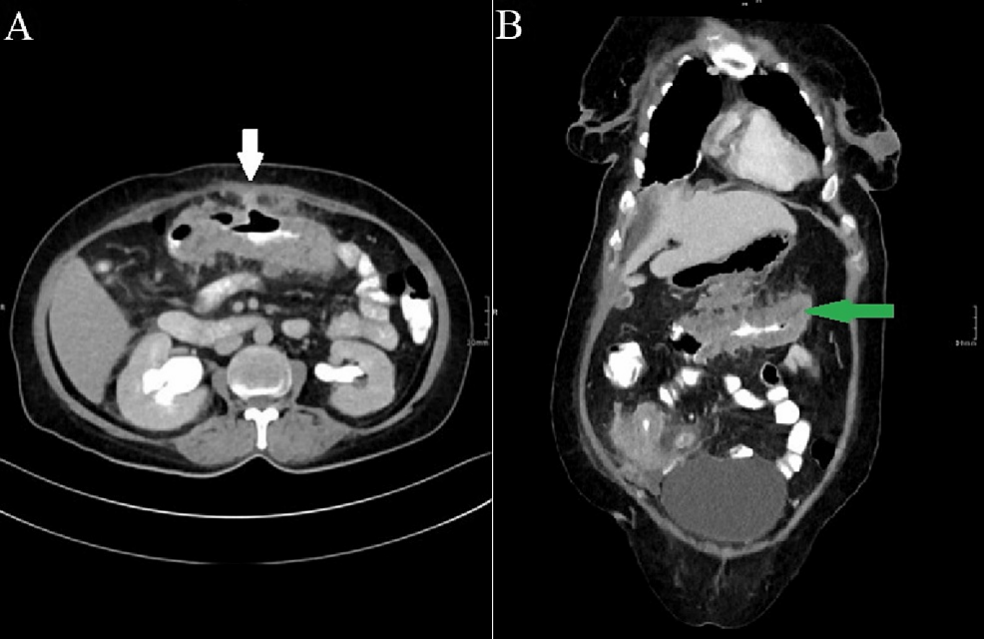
CT scan of the abdomen - (A) axial view showing circumferential mural wall thickening (white arrow). (B) Sagittal view showing circumferential mural wall thickening of the colon (green arrow)
